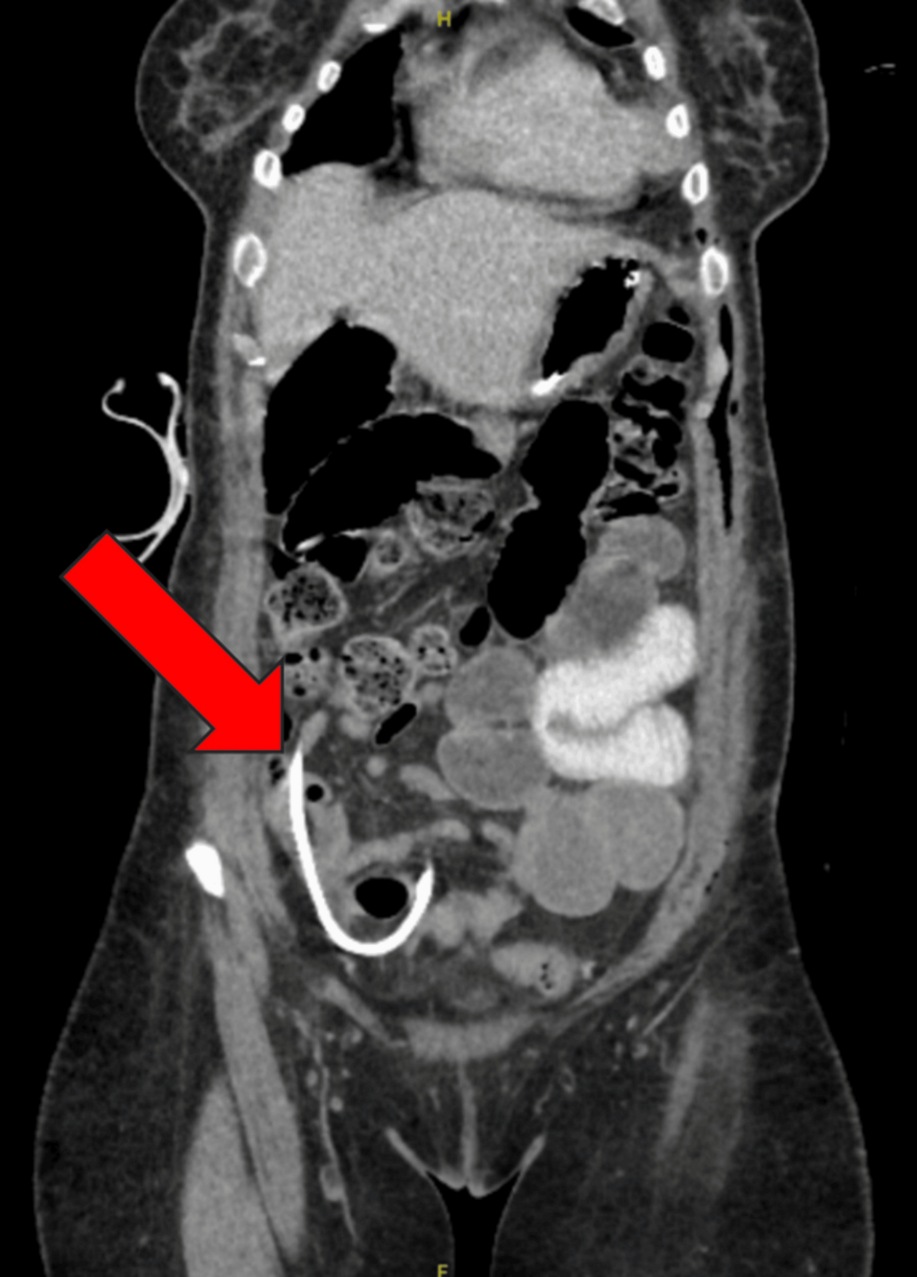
CT abdomen and pelvis with PO contrast, revealing small bowel dilation with a transition point in the right lower quadrant with associated mesenteric swirling. PO: per os (oral) Arrow size: 3.2 cm x 0.8 cm
